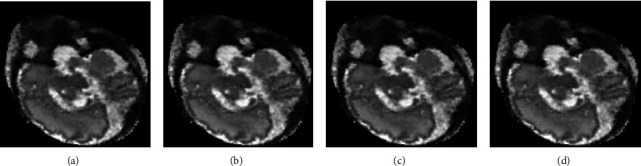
Reconstructed results of the MRI data with different regularization parameters *λ*: (a) *λ* = 0.5, (b) *λ* = 1.0, (c) *λ* = 1.5, and (d) *λ* = 2.0.
